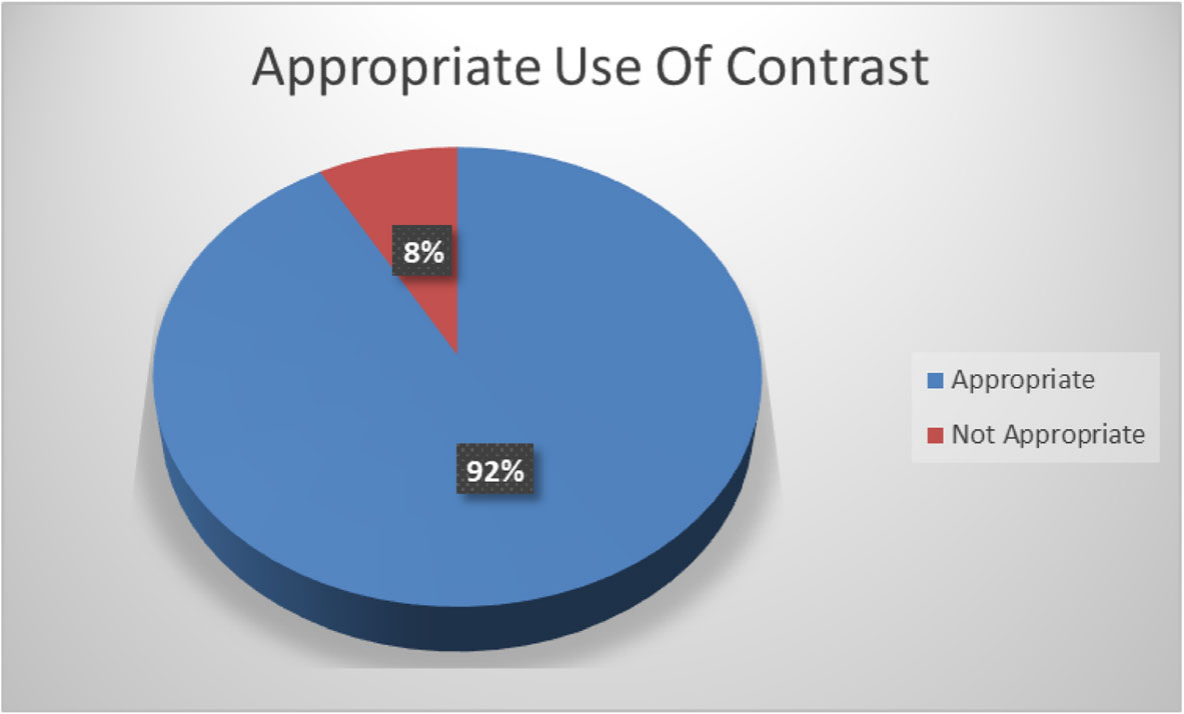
Appropriate Use of Contrast. Review of images by Cardiologist showed that 92% of studies receiving contrast in a sonographer driven protocol were appropriate
